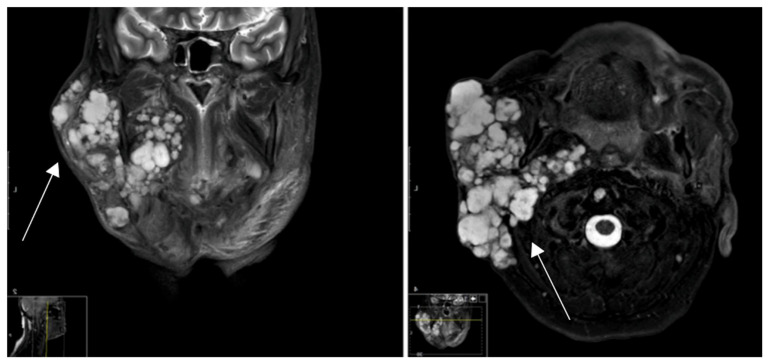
MRI. Multilocular local tumor recurrence and extra parotid tumor spread of a pleomorphic adenoma by status post-R1-resection many years earlier.
